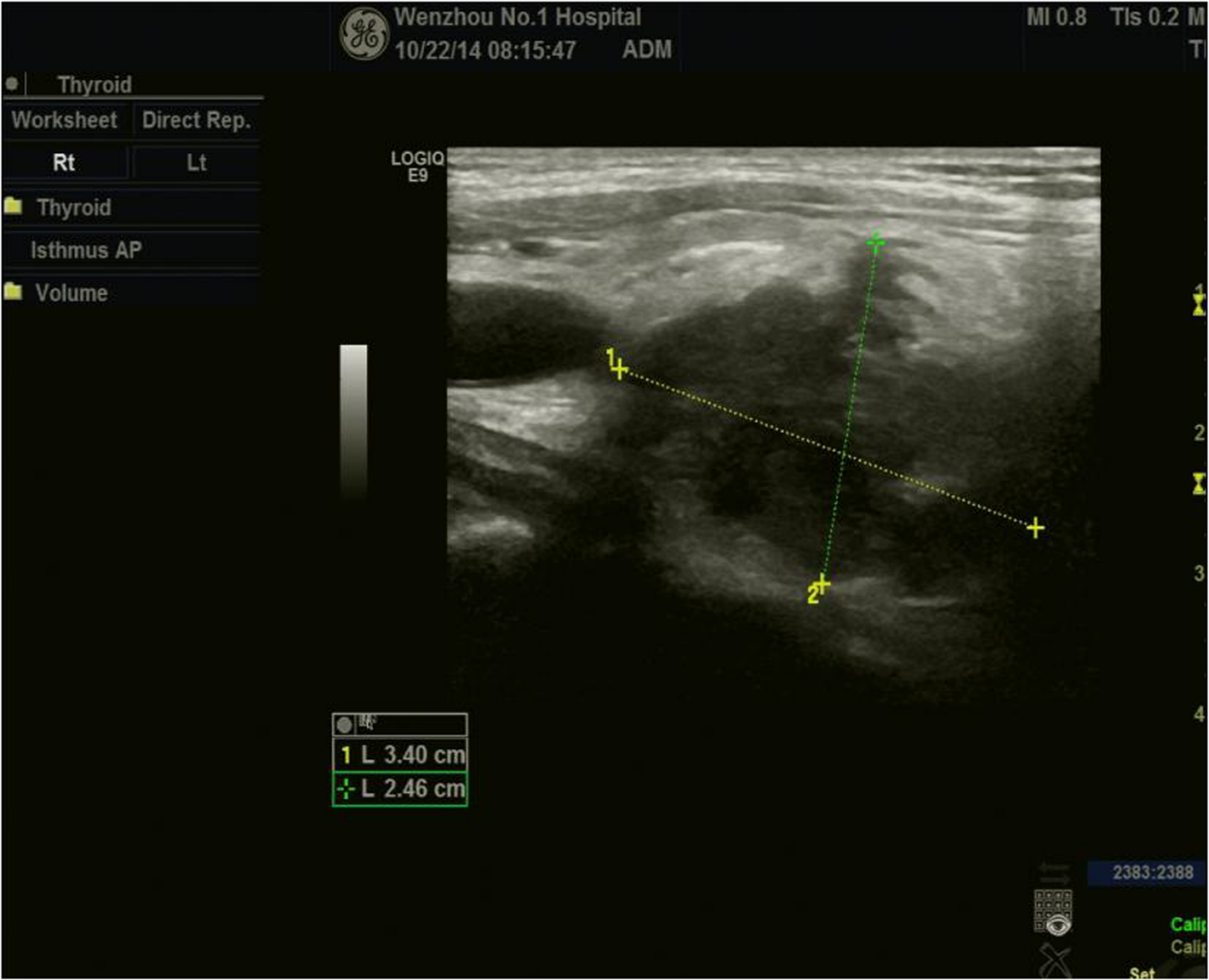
Ultrasound reexamination in a week before death showed a solitary, irregular, hypoechoic mass with some hyperechoic foci. Its border was unclear and the max diameter was 3.4 cm.
